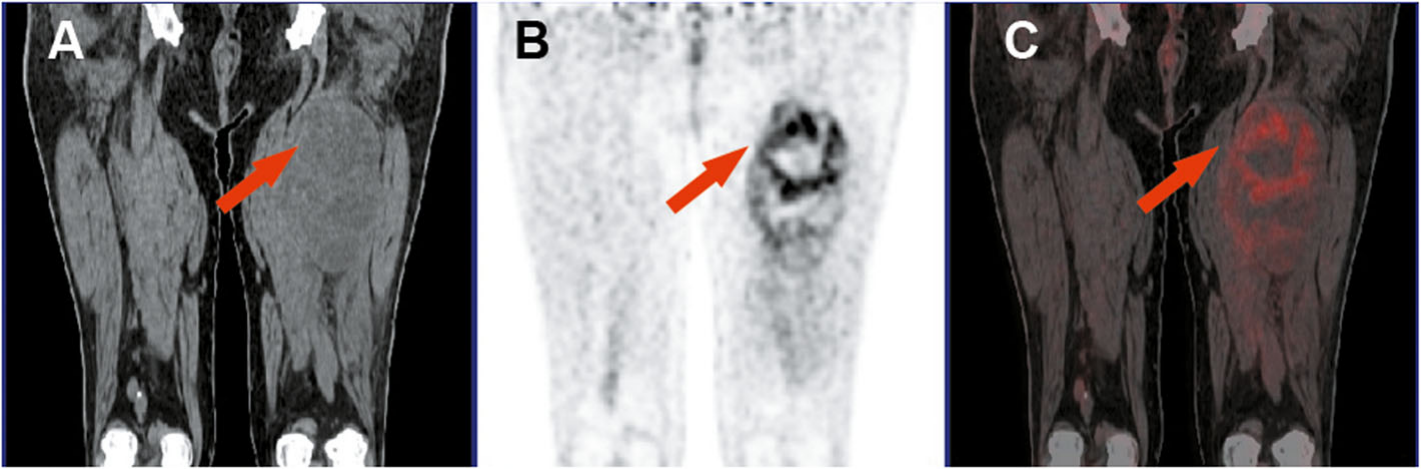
Liposarcoma of the left thigh. (a) CT-coronal plane. (b) PET-coronal plane. (c) PET/CT fusion image. As indicated by the red arrow, the density of the mass was equal or slightly lower than that of the adjacent muscle tissue. Moreover, the uptake of ^18^F-FDG increased significantly and heterogeneously. Tumor size = 16.9 cm, SUVmax = 8.3, HF = 2.93, TLG = 519.1, MTV = 123.6 cm^3^, and *P* value = 0.99
